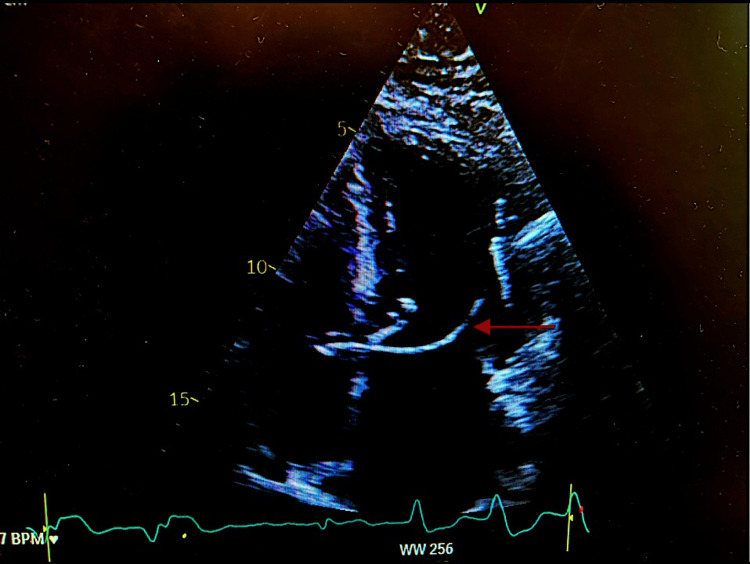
Four chamber view on transthoracic echocardiogram. Red arrow points to the temporary pacing lead traversing the interatrial septum and entering the left ventricle.
